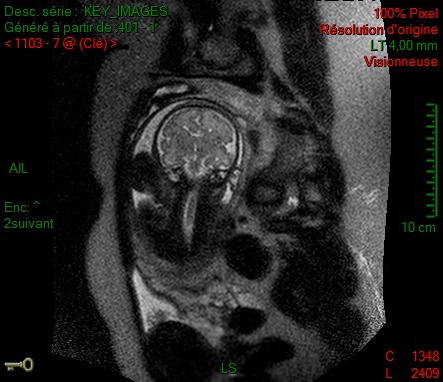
T2‐weighted MRI at 32 WG, coronal view showing perisylvian microgyria on the right side
